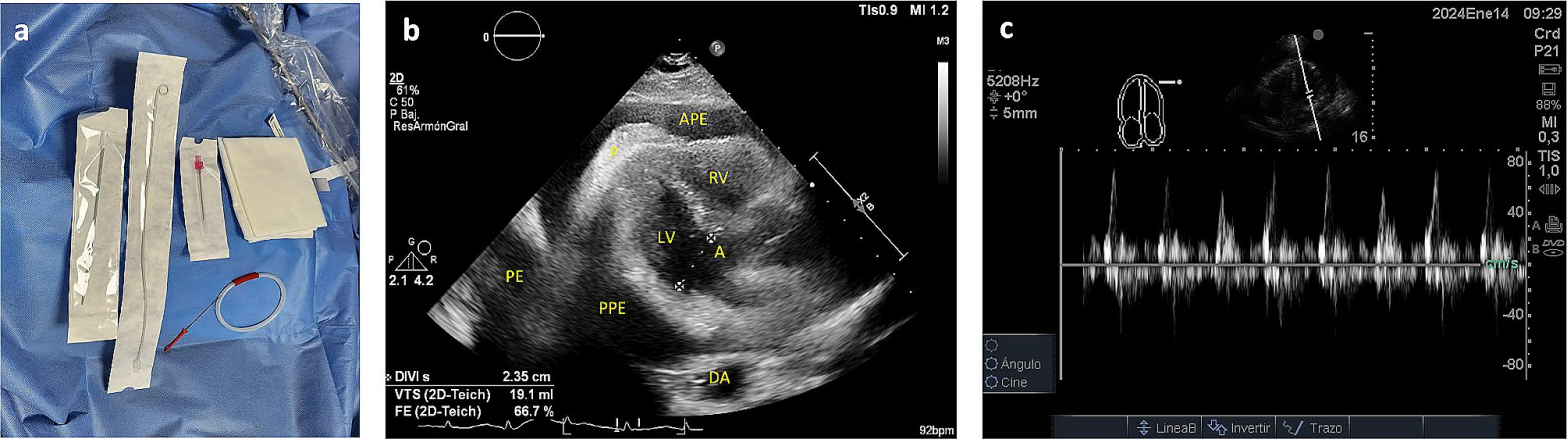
(a) Pericardiocentesis kit (b) Long axis window showing anterior and posterior pericardial effusion. APE: Anterior pericardial effusion, RV: right ventricle, LV: left ventricle, A: aorta, PE: pleural effusion, PPE: posterior pericardial effusion, DA: descending aorta, P: pericardium thickness (c) Doppler through mitral valve showing changes in peak E-wave (> 25%) during respiration
